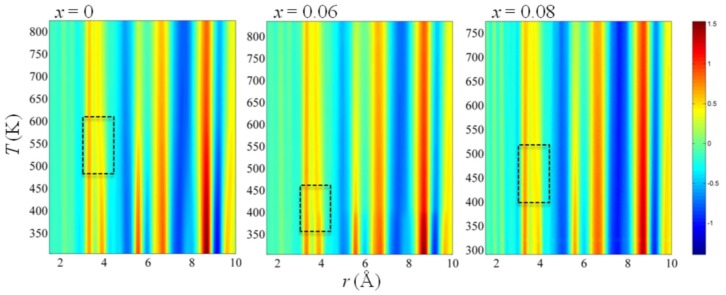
Temperature evolution of X-ray PDF intensities for BNBT for *x* = 0, 0.06, and 0.08; the dashed rectangles mark the temperature ranges of the most pronounced changes in PDFs. Reproduced with permission from [39].
